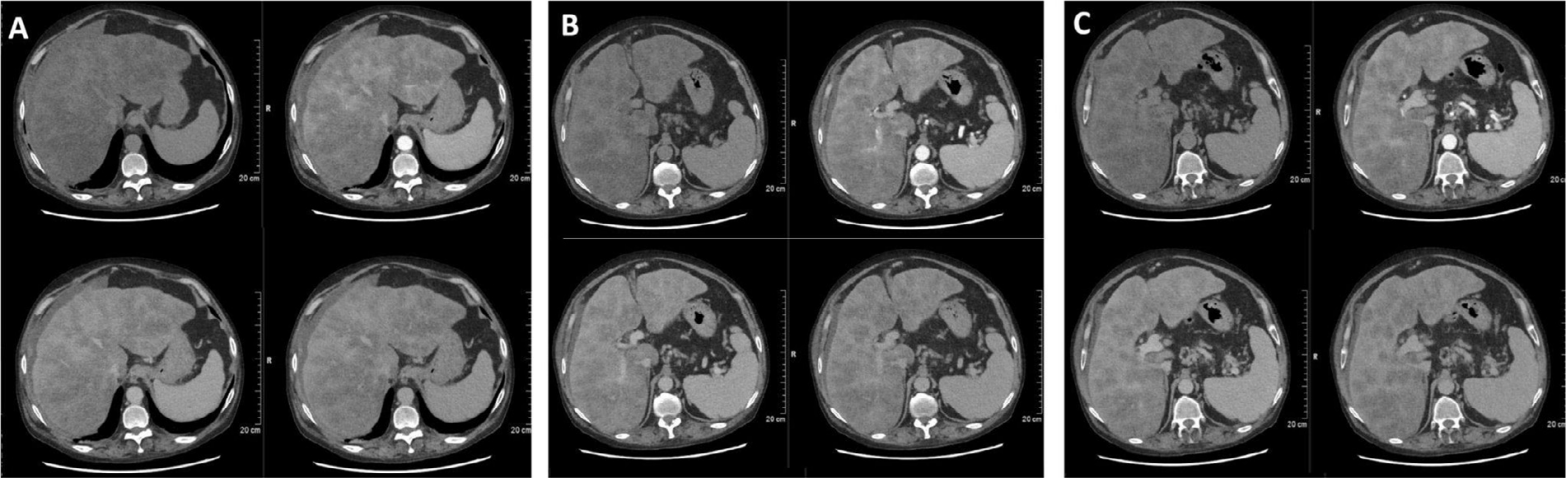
unenhanced and contrast-enhanced CT axial scans at different levels (A-C) showing multiple hypo-attenuating lesions spread throughout the liver, which appears hypertrophic with irregular margins.
